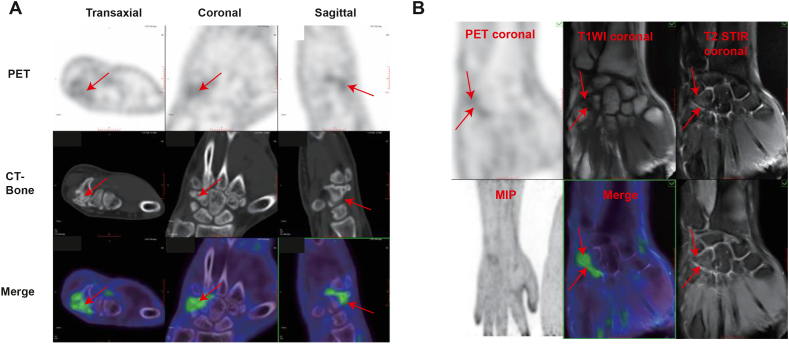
Clinical Case: ^18^F-Pentixafor-based PET scans assist in evaluating hamulus fracture healing in a young patient (A) ^18^F-Pentixafor PET-CT results of an 18-year-old female patient suffering hamulus fracture 6 months after a right palm injury (after 6 months), showing ^18^F-Pentixafor uptake in fracture line and callus area. Scale bar = 10 mm. (B) ^18^F-Pentixafor PET-MR results of the same patient, showing ^18^F-Pentixafor uptake in fractured bone with healed cortical bone and bone marrow edema (T1WI: T1-weighted imaging, STIR: Short Time of Inversion Recovery, MIP: Maximum Intensity Projection). Scale bar = 10 mm.
